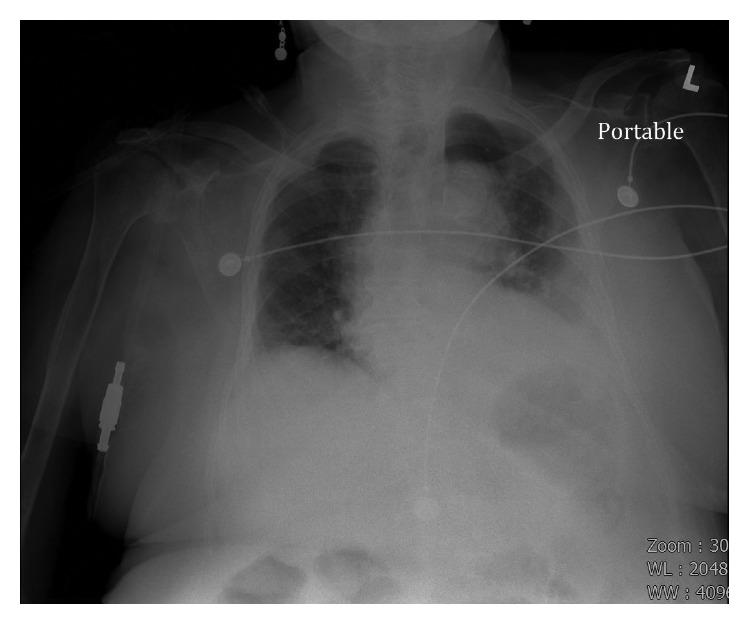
Chest X-ray obtained on admission showing left lower lung infiltrate, with blunting of the adjacent costophrenic angle consistent with a pleural effusion.
